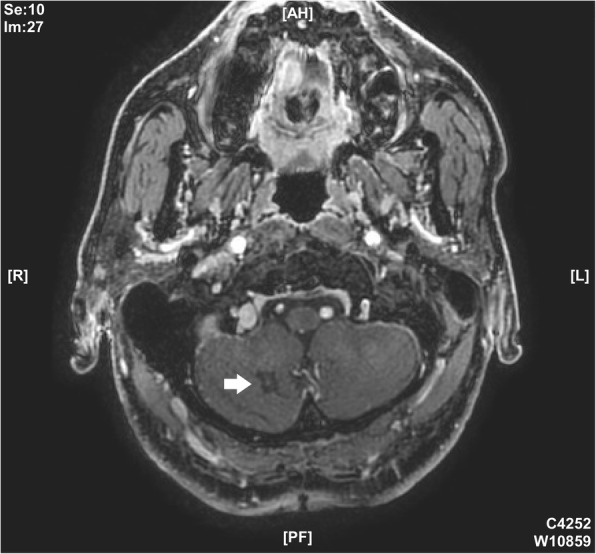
MRI image of cerebellar abscess resolution after surgery.MRI image showed that abscess in the right cerebellum was almost completely resolved 3 months after surgery. The arrow indicated the abscesses that has not resolved
